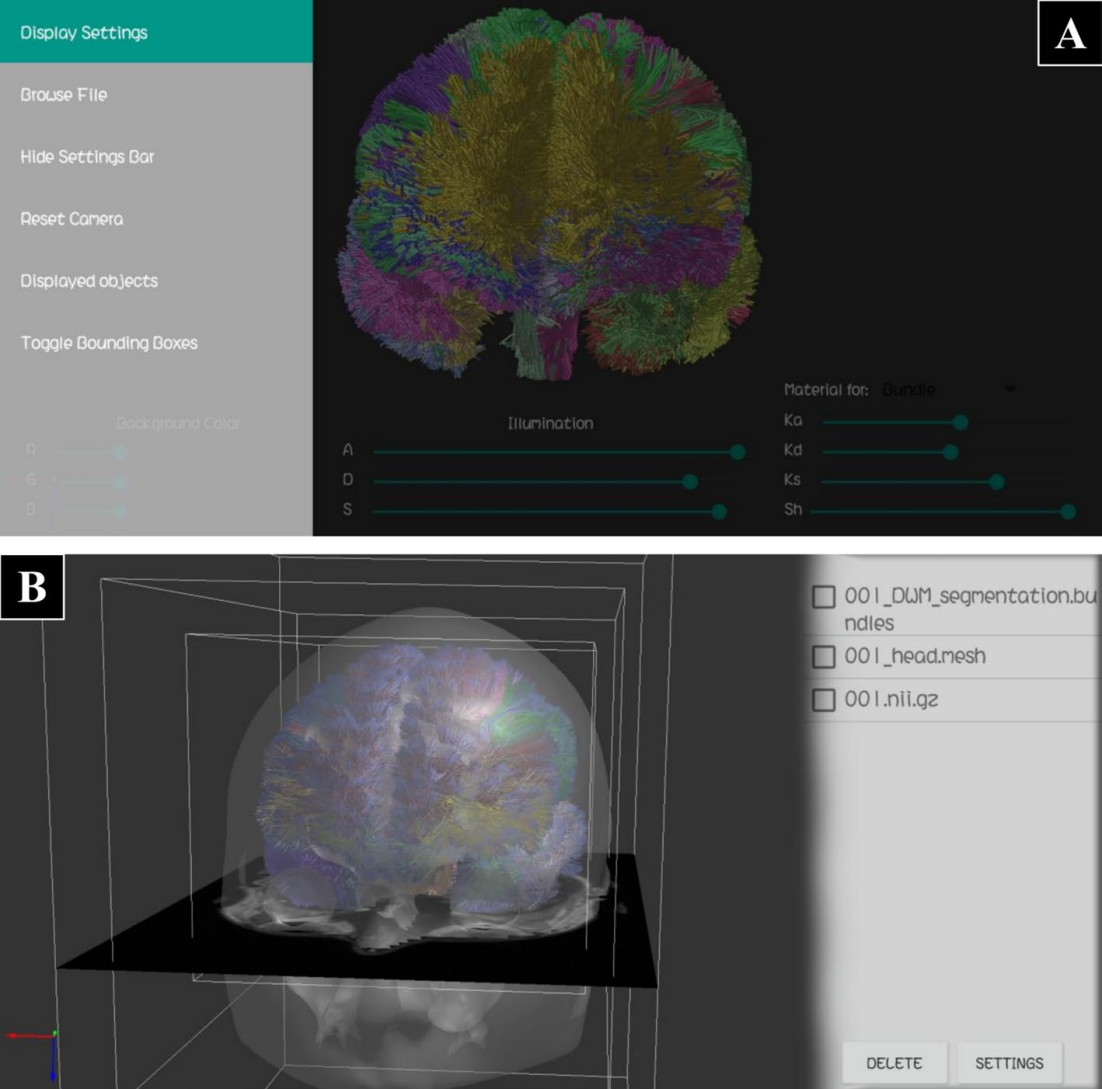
Main components of the application interface. A Main screen with the “Settings Bar” visible. When this bar is displayed, the main visualization window and the other setting bars are disabled and obscured. In the main visualization screen a tractography is displayed, as well as the “Display Settings Bar”. B Main screen with the “Object Settings Bar” visible, listing the three objects loaded: a tractography dataset, a head mesh and a slice from an MRI volume. Multiple objects can be selected for removing them. Only one object must be selected for displaying its settings options on the bar. In the main visualization screen, the three objects are displayed with their bounding boxes
